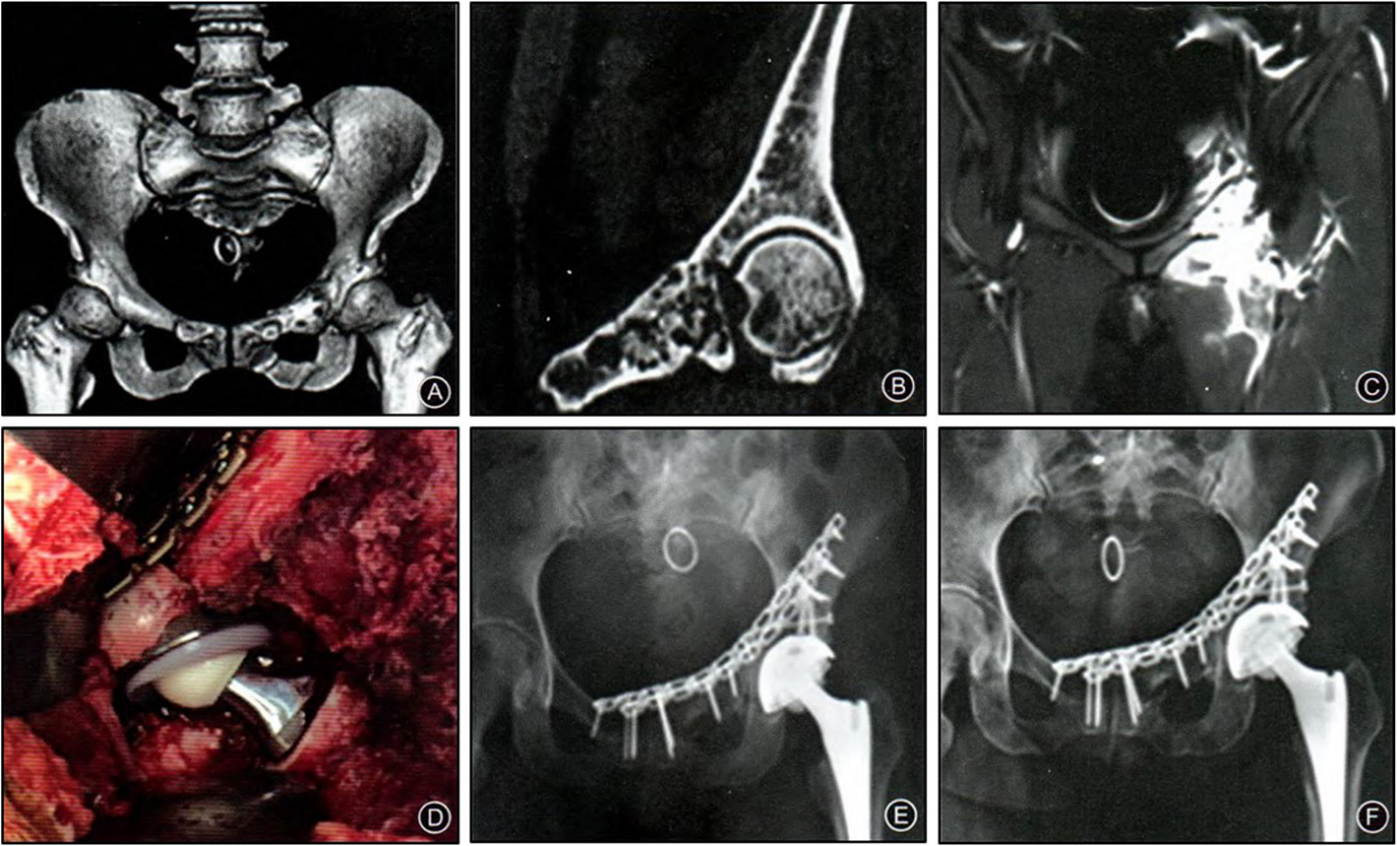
A 56y female patient diagnosed with Type A tumor (chondrosarcoma). a. Preoperative three-dimensional CT shows left pubic ramus tumor with anterior edge of acetabulum involved; b. Preoperative coronal CT shows pubic ramus tumor with anterior edge of acetabulum involved; c. Preoperative MRI T2 W1 shows enhanced signal on the pubic ramus and anterior edge of acetabulum; d. Intraoperative autogenous femoral head implantation and reconstruction of the acetabulum; e. Postoperative X-ray shows the reconstruction and prosthetic position; f: 1y postoperative pelvic X-ray shows the reconstruction and prosthetic position
